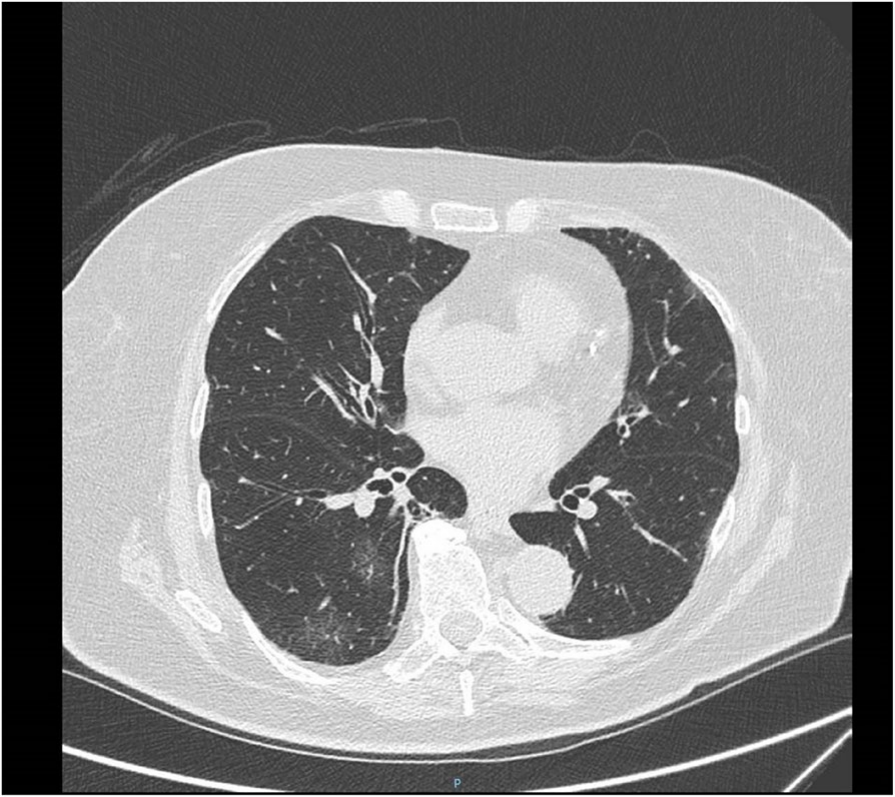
The first HRCT which shows mild pneumonia signs
